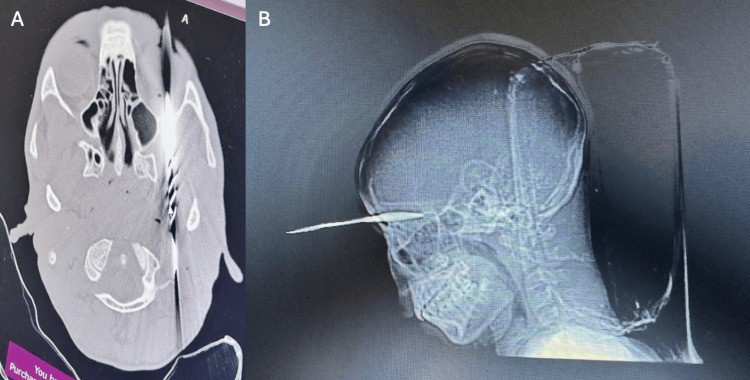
(A) Axial view and (B) sagittal view of the CT scan showing the arrowhead CT: computed tomography
